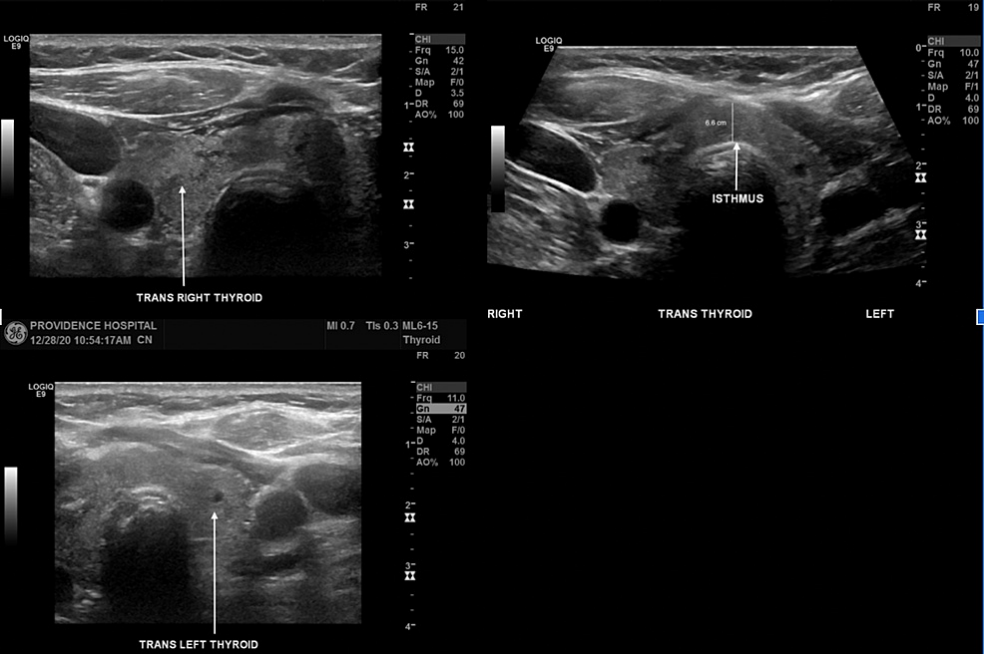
Thyroid ultrasound showing mildly heterogeneous gland with thickened isthmus without a discrete suspicious thyroid nodule
